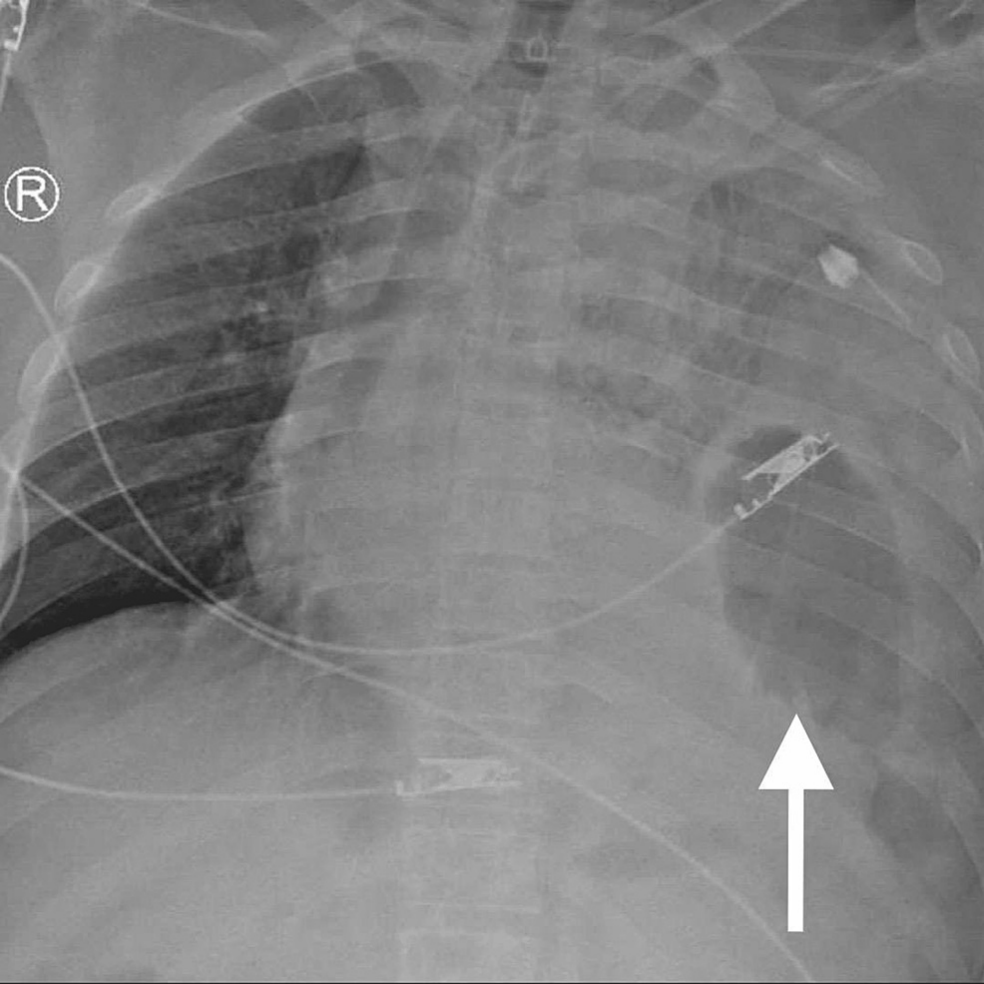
Chest X-ray showing a mediastinal shift towards the right with multiple left rib fractures, and opacities in the left thorax.
